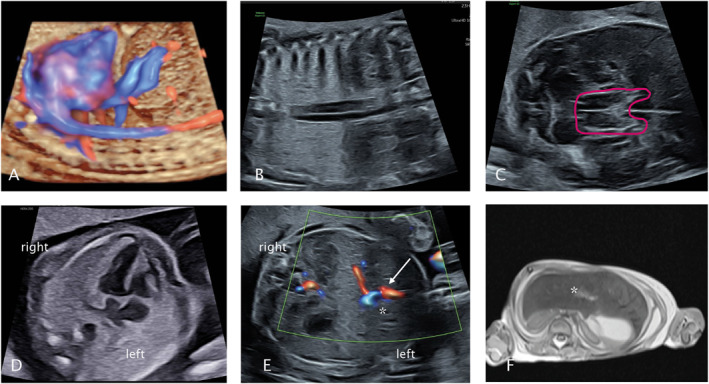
Sonographic images of the fetus in 22 gestational weeks showing azygos continuation of the inferior vena cava (A + B), molar tooth sign (C) and left isomerism with dextrocardia (D), a median located gallbladder (*) and persistent right umbilical vein (↑, E). The post mortem MRI (F) confirmed left isomerism with a horizontally oriented liver (*) and showed gallbladder aplasia and a hypoplastic spleen.
